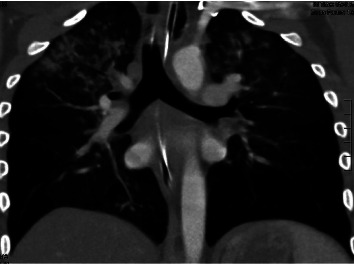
Coronal view of CT chest scan showing diffuse alveolar ground-glass and nodular opacities prior to initiation of diuretic therapy.
